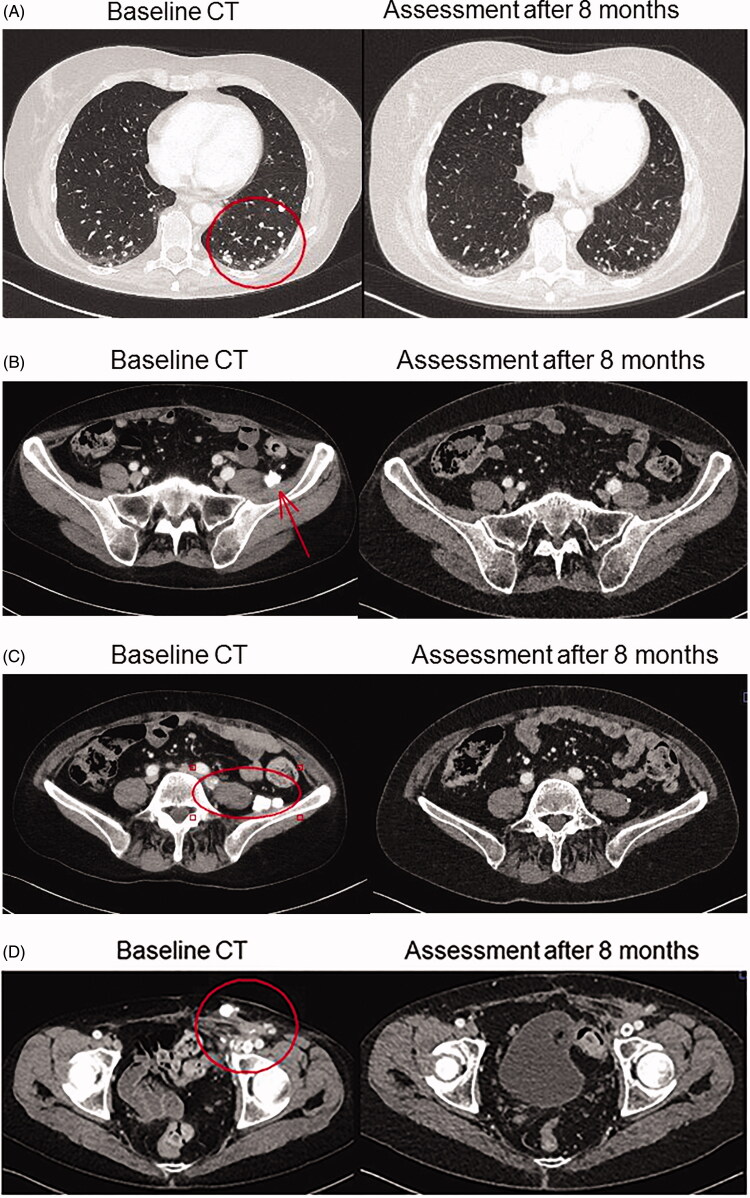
Baseline and eight months CT scans demonstrating complete response for a patient with low-grade serous ovarian cancer treated with combination of BRAF and MEK inhibitors. (A) Lung metastases. (B, C) Lymph node metastases at left pelvic wall. (D) Lymph node metastases in left groin.
